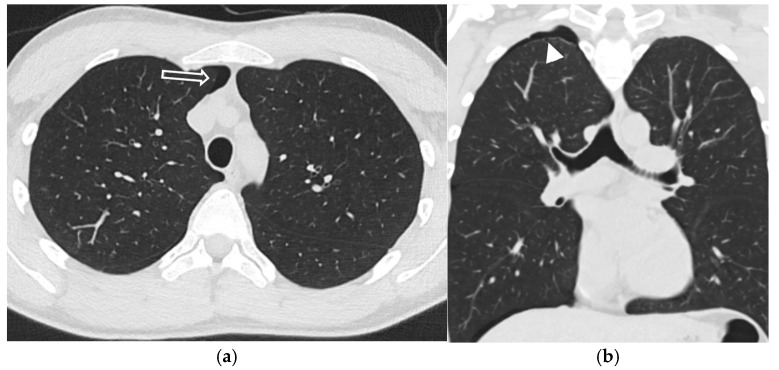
19-year-old male with a history of IgA nephropathy and vasculitis presents with chest pain. Axial and coronal CT images in lung window (a,b) demonstrate a small right pneumothorax (open white arrow). The coronal image also shows small right apical blebs (white arrowhead).
